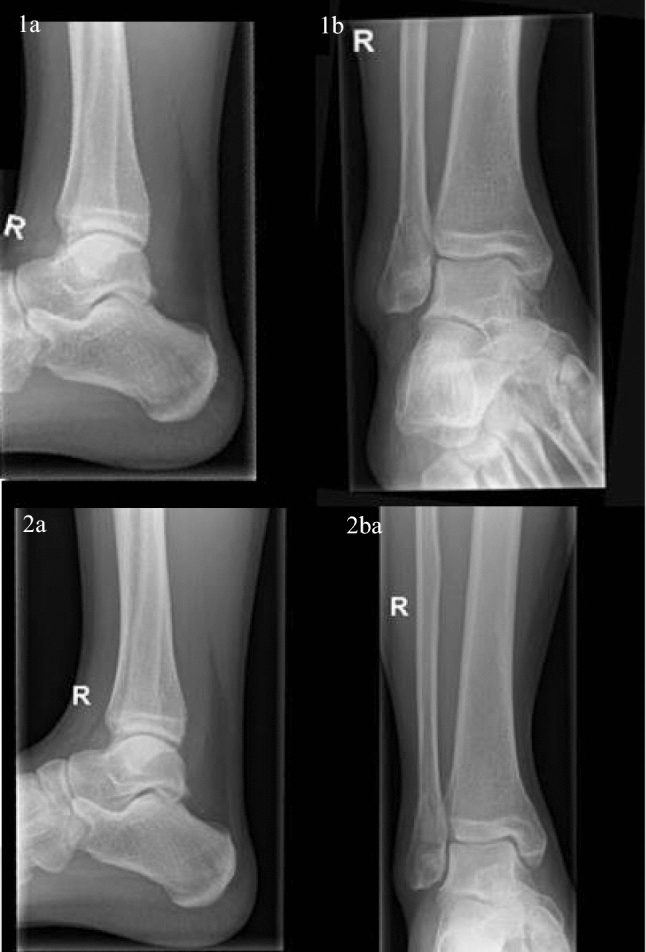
Radiographs taken posttraumatic and after six weeks of permissive weightbearing. Image 1a and b were obtained 2 days after trauma for this patients. Lateral view (image 1a) of ankle shows no evident signs of fracturing. Mortise (image 1b) view shows an evident fracture without widening of the medial clear space. Image 2a & b were obtained 6 weeks after permissive weightbearing. Lateral view (image 2a) shows no signs of fracture displacement. Mortise view (image 2b) shows no signs of fracture displacement and show signs of callus bridging
